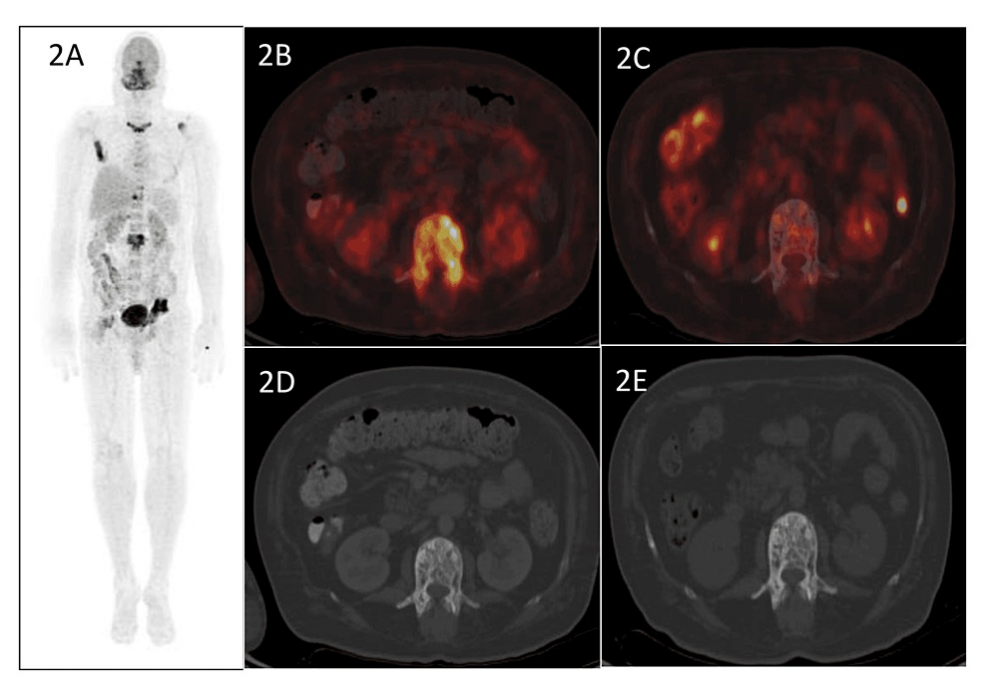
18F-FDG PET/CT (A) Fluorodeoxyglucose (FDG) PET/CT shows multiple abnormal foci of tracer uptake in the regions of the skull, sternum, right scapula, a few vertebrae, region of left shoulder, left hemipelvis, and right femur. (B and D) Trans-axial fused PET/CT and CT images localize the tracer uptake to lytic sclerotic changes in the L2 vertebra (SUV max – 11.1). (C and E) A regional FDG PET/CT done following zoledronate infusion shows the resolution of FDG uptake in the L2 vertebra on the PET/CT images with no significant anatomical change on the CT images.
